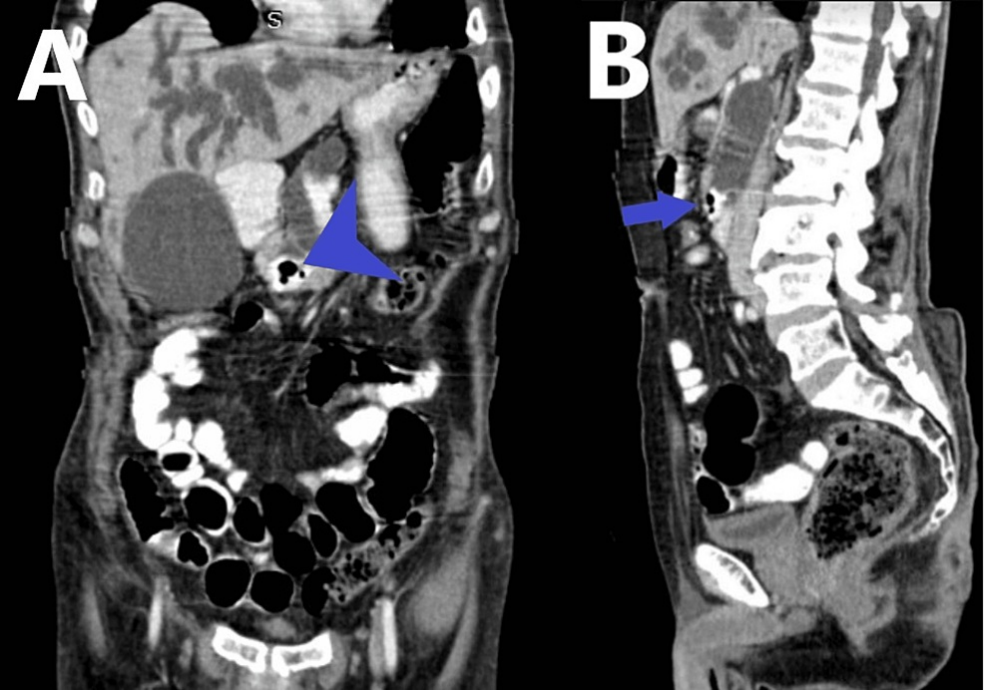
Coronal (A) and sagittal (B) oral and intravenous contrast enhanced multiplanar reformatted computed tomographic images of abdomen showing diverticulum (arrowhead and arrow)
